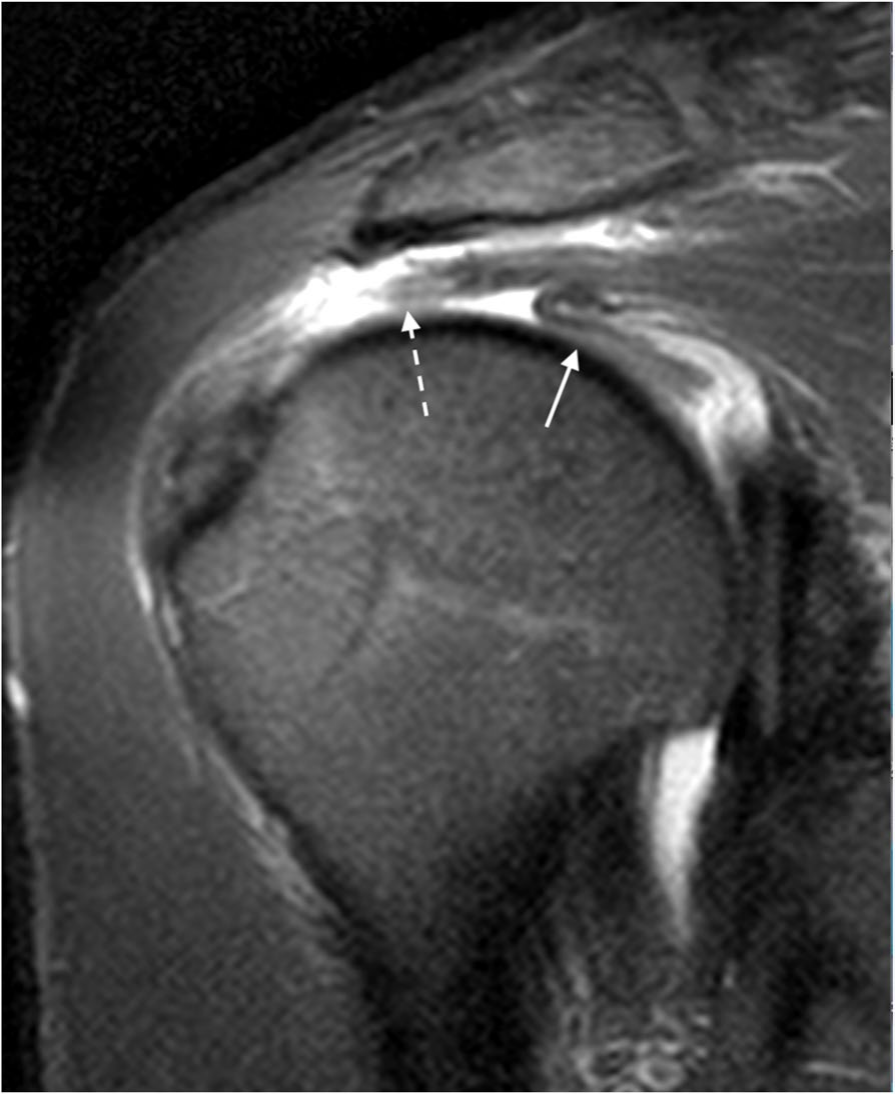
Full thickness delaminated tear of the supraspinatus tendon with articular layer flipped medially into the articular space. Oblique coronal fat-suppressed PD-weighted MRI reveals a complete SS tendon tear (dashed arrow) and note how the articular layer is flipped downwards into the articular space (arrow)
